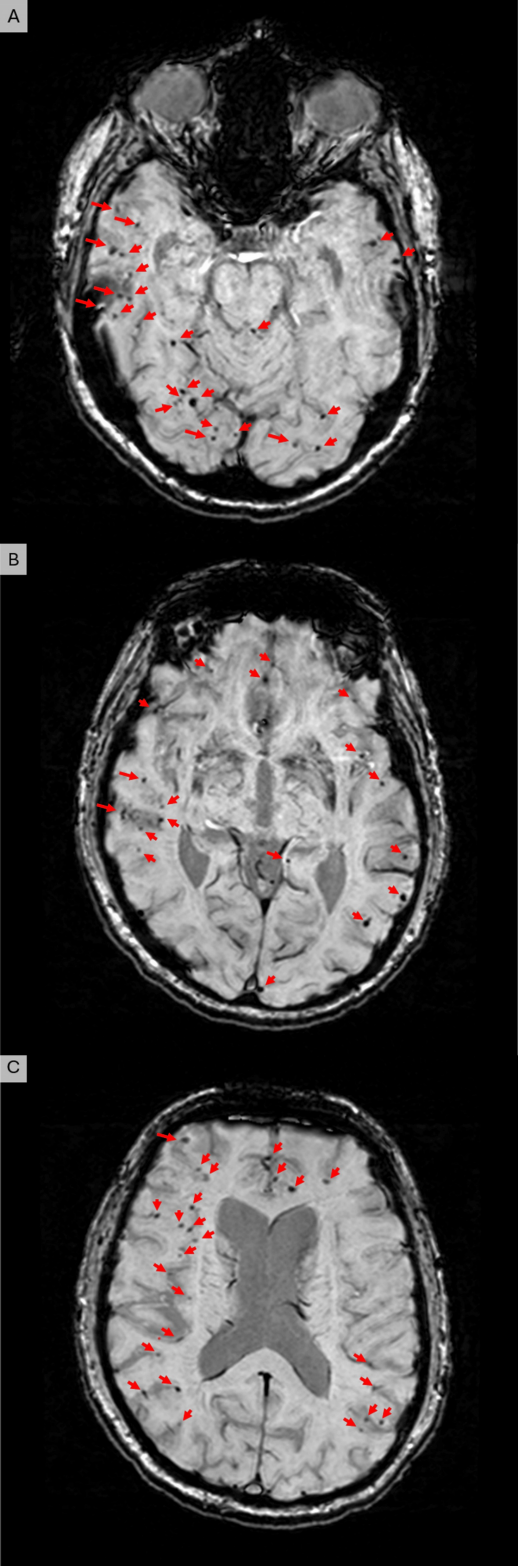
MR images obtained with a susceptibility-weighted imaging sequence Images obtained from the level of the midbrain, inferiorly (A) through the basal ganglia (B), to the corona radiata superiorly (C). The images demonstrate innumerable microhemorrhages (red arrows) throughout both cerebral hemispheres in a predominantly lobar distribution, consistent with the diagnosis of cerebral amyloid angiopathy. Red arrows indicate microhemorrhages.
